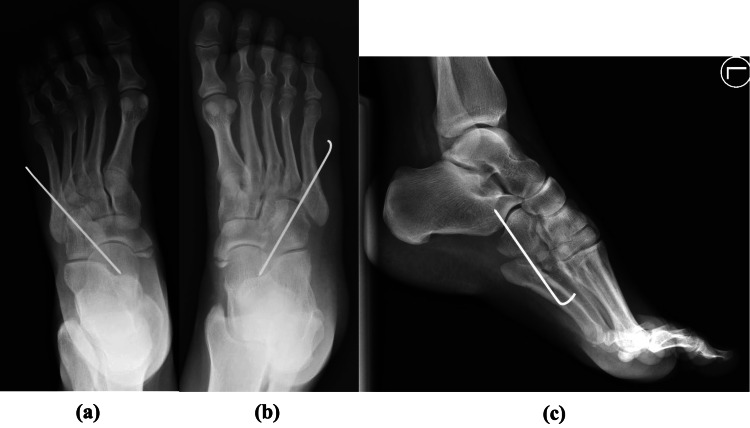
Post-operative anteroposterior (a), oblique (b), and lateral (c) radiographs demonstrating pinning of the fifth TMT joint with reduction of the cuboid. TMT, tarsometatarsal
